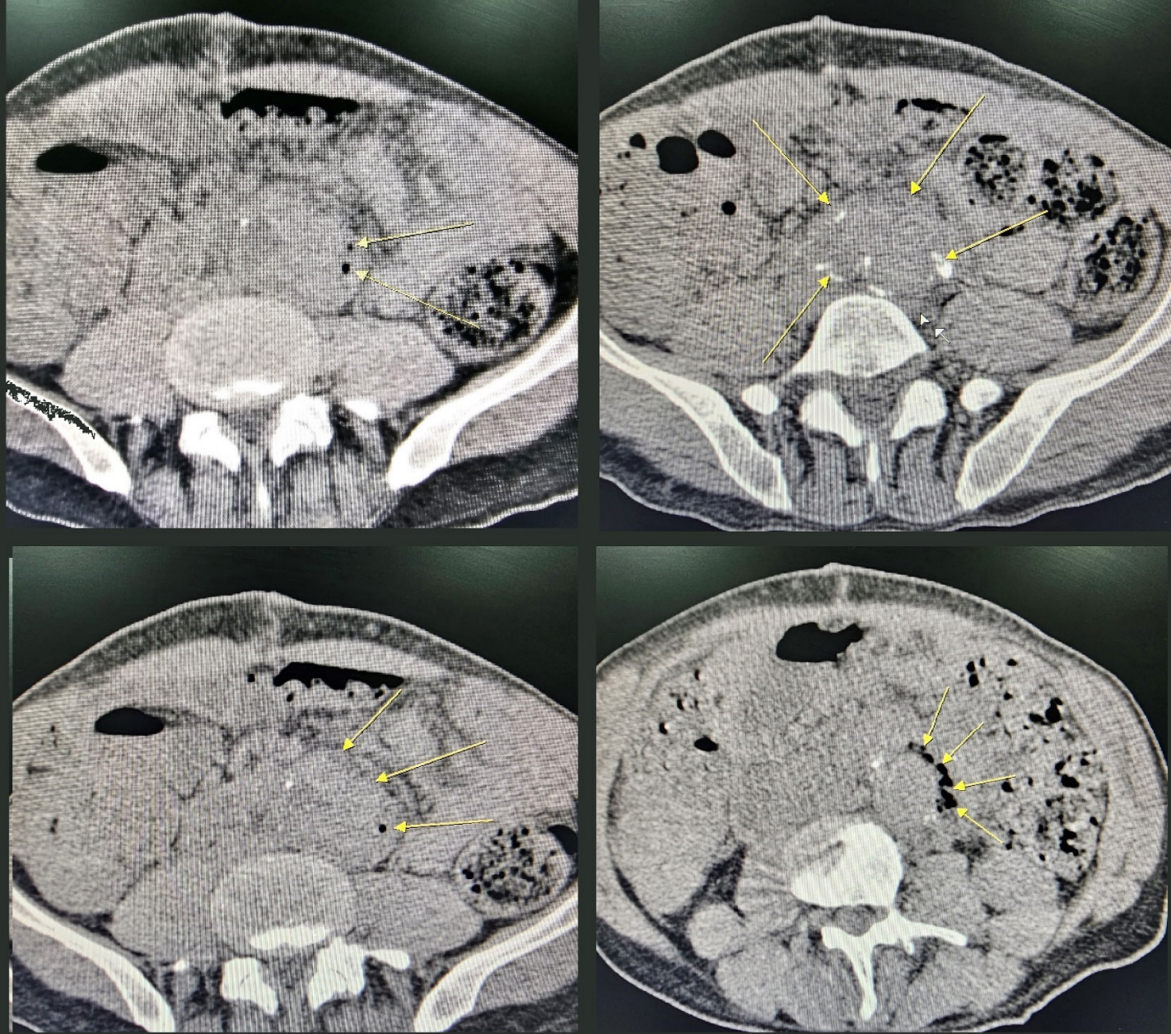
Computed tomography scan without contrast demonstrating infected aneurysm suspicious to have air bubbles in its wall. (Arrows indicate air bubbles (black spaces) and the aneurysm).
